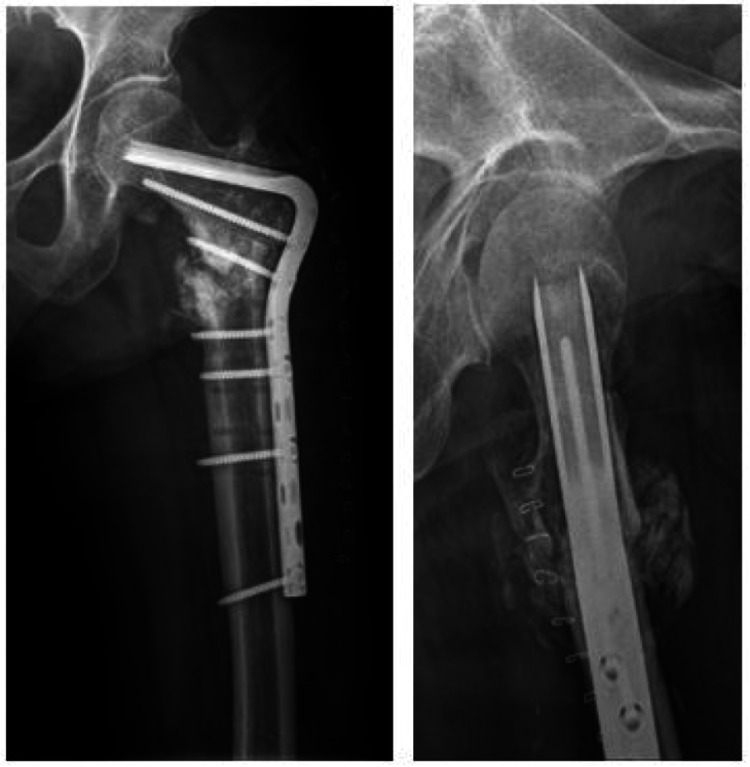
Radiographs after re-revision surgery shows corrected varus alignment and abundant bone chips which was made by decortication and autogenous cancellous bone graft.
